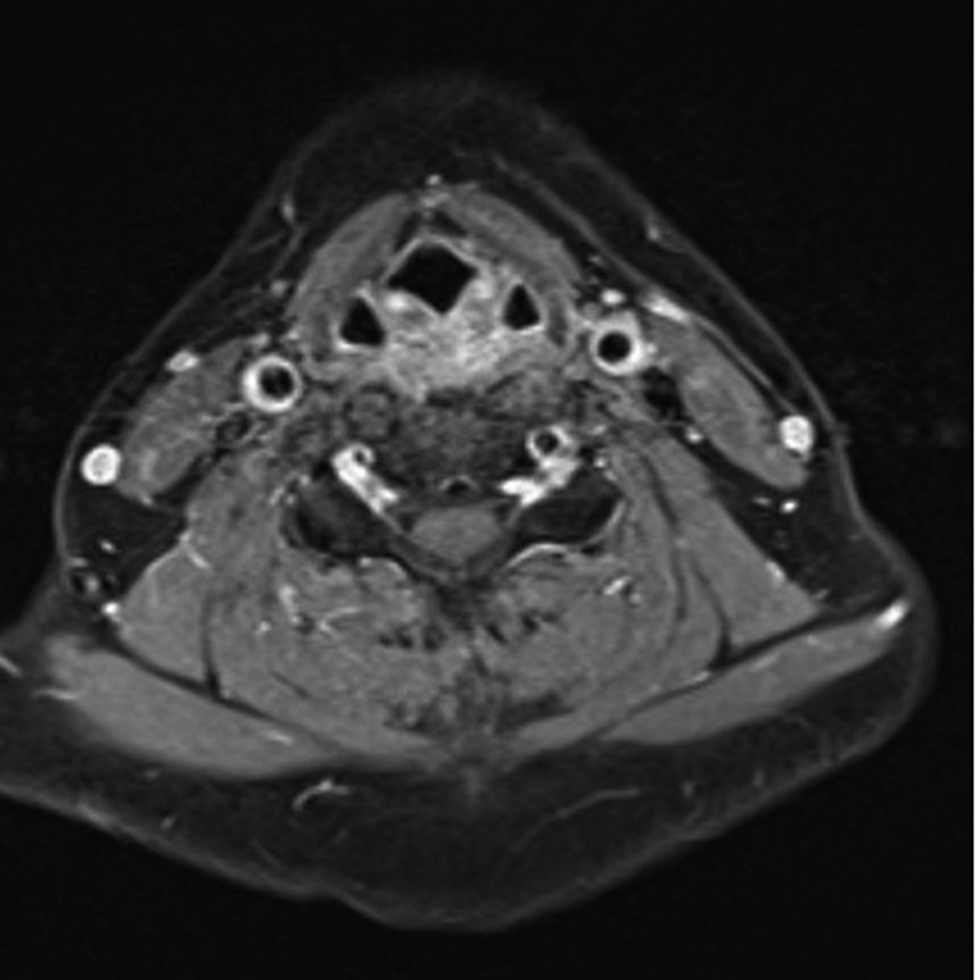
Magnetic resonance imaging at follow-up.
